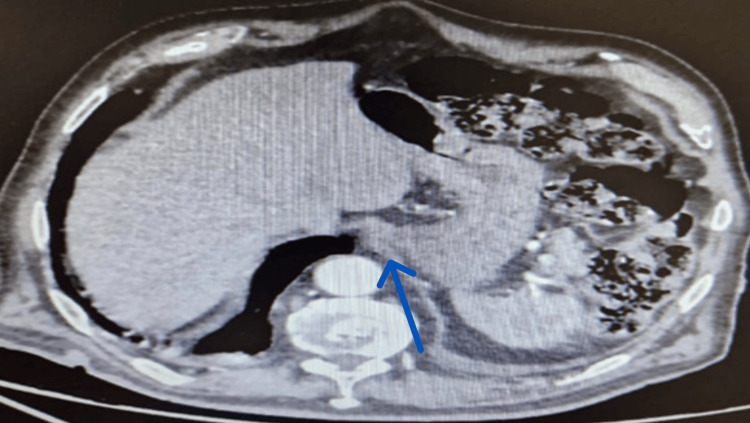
Contrast-enhanced abdominal CT scan does not show any extravasation of the contrast agent or signs of active bleeding
